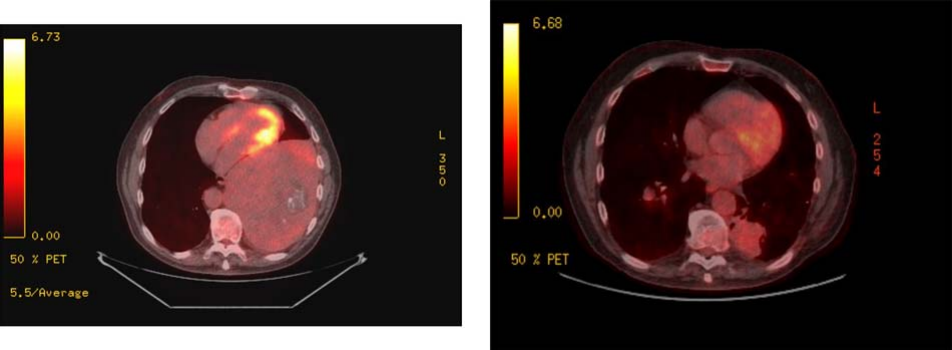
PET scans; large left-sided pleural-based tumour exhibits mild FDG uptake, with the SUV max of 2.9 (left); recurrent disease at the pulmonary resection margin along with contralateral pulmonary metastases demonstrated (right).
